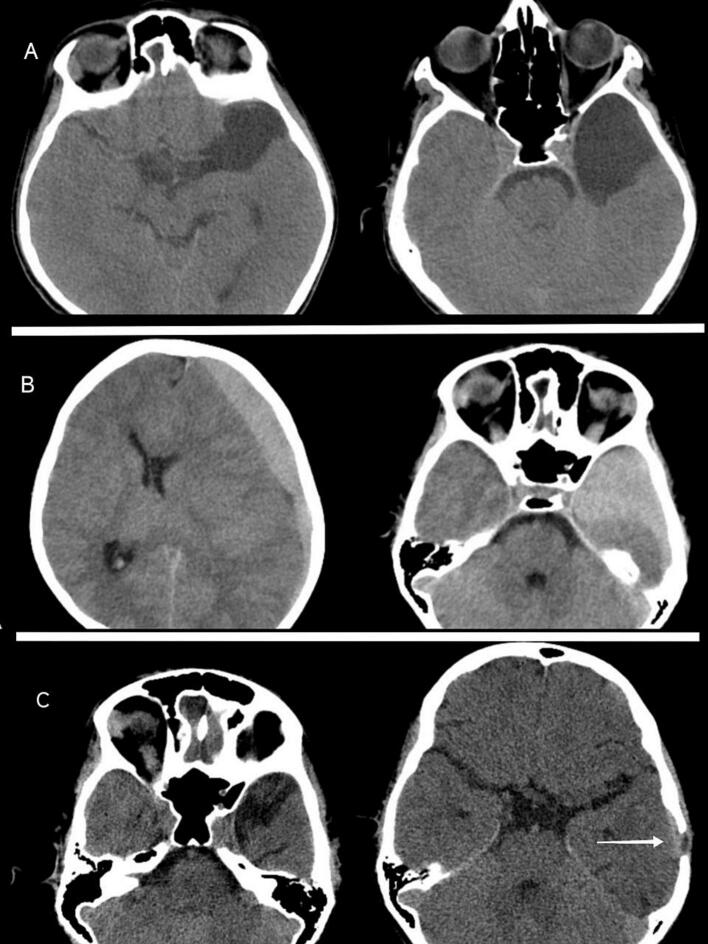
A) Initial CT: This axial non-contrast CT scan shows a hypodense, well-circumscribed lesion in the left middle cranial fossa, consistent with an arachnoid cyst (Galassi type II). No signs of acute hemorrhage, mass effect, or midline shift are present. The patient presented in a neurologically normal condition. B) Acute Deterioration, Hemorrhagic Complication: The previously hypodense arachnoid cyst is now partially hyperdense, indicating intracystic hemorrhage, a hyperdense crescent-shaped collection overlying the left cerebral hemisphere, consistent with a subacute subdural hematoma. A mass effect and midline shift are visible, including compression of adjacent brain structures. C) Postoperative CT: This three-month postoperative scan shows evacuation of both the intracystic hemorrhage and the subdural hematoma. The arachnoid cyst cavity appears decompressed. The brain parenchyma is relaxed, with re-expansion of the adjacent structures. No residual mass effect or new hemorrhage is noted, and the site of the burr hole.
